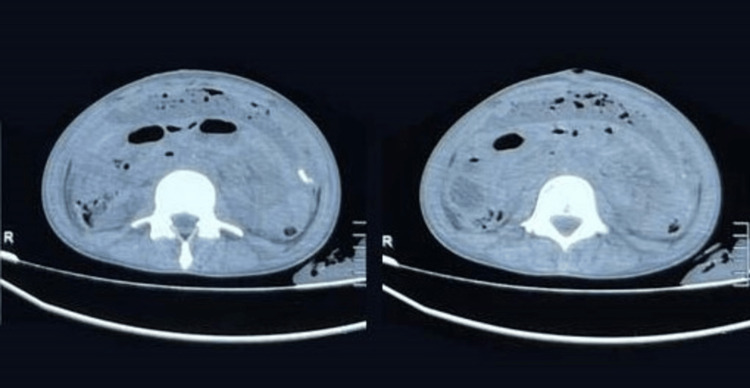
CT of the abdomen showing moderate ascites with multiple air pockets in the peritoneal cavity
